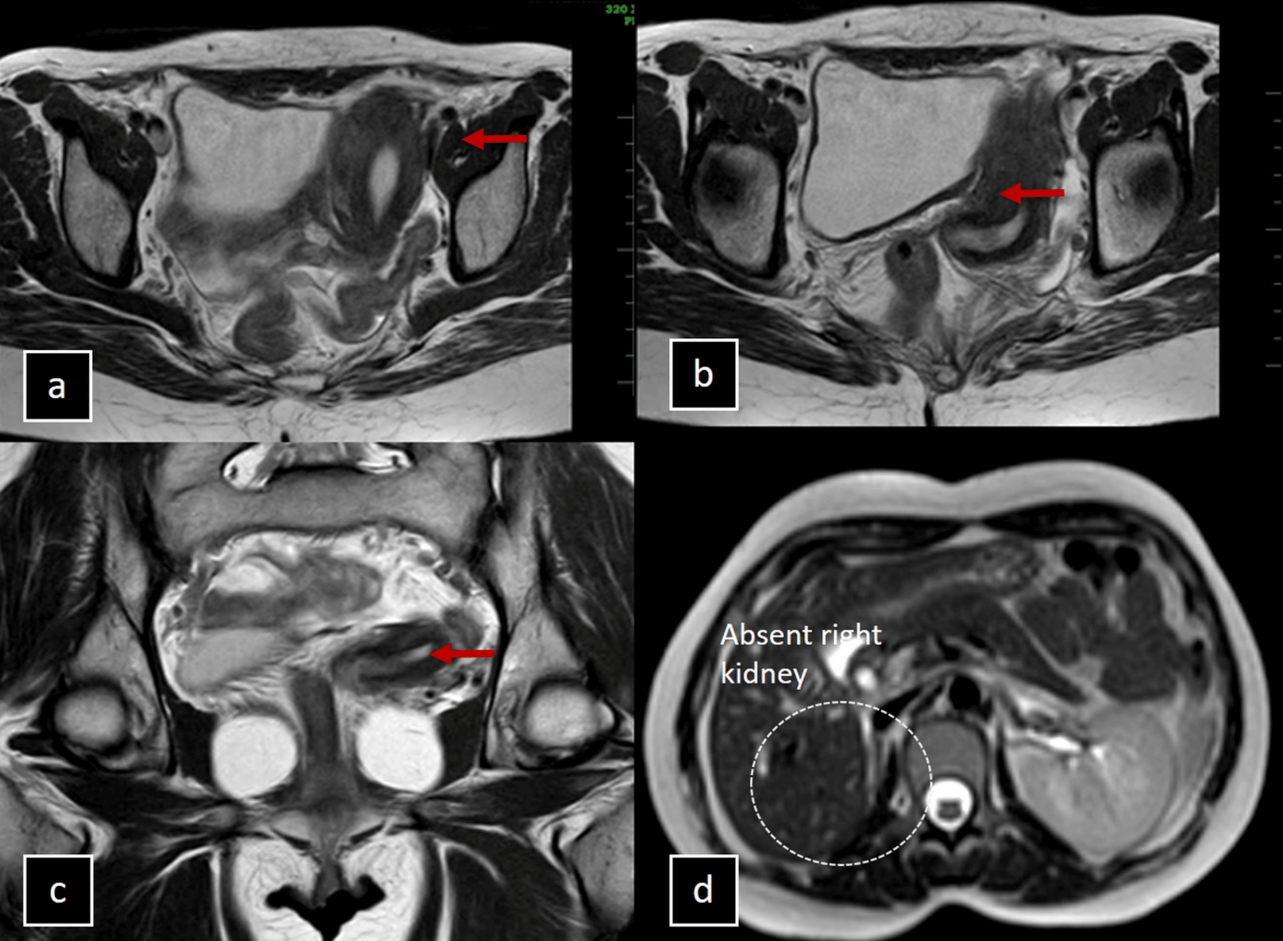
(a-d) Imaging of the True Unicornuate Uterus with No Rudimentary Horn in a 29-Year-Old Female. MRI images of a 29-year-old female. T2 axial sections (a, b), coronal section (c), and T2 axial section at the level of the bilateral kidneys show a uterus displaced to the left adnexa with no evidence of a rudimentary horn (red arrows) and an absent right ovary. Additionally, there is an absence of the right kidney (d). These findings are consistent with a Type IId True Unicornuate (Left) uterus with no rudimentary horn.
